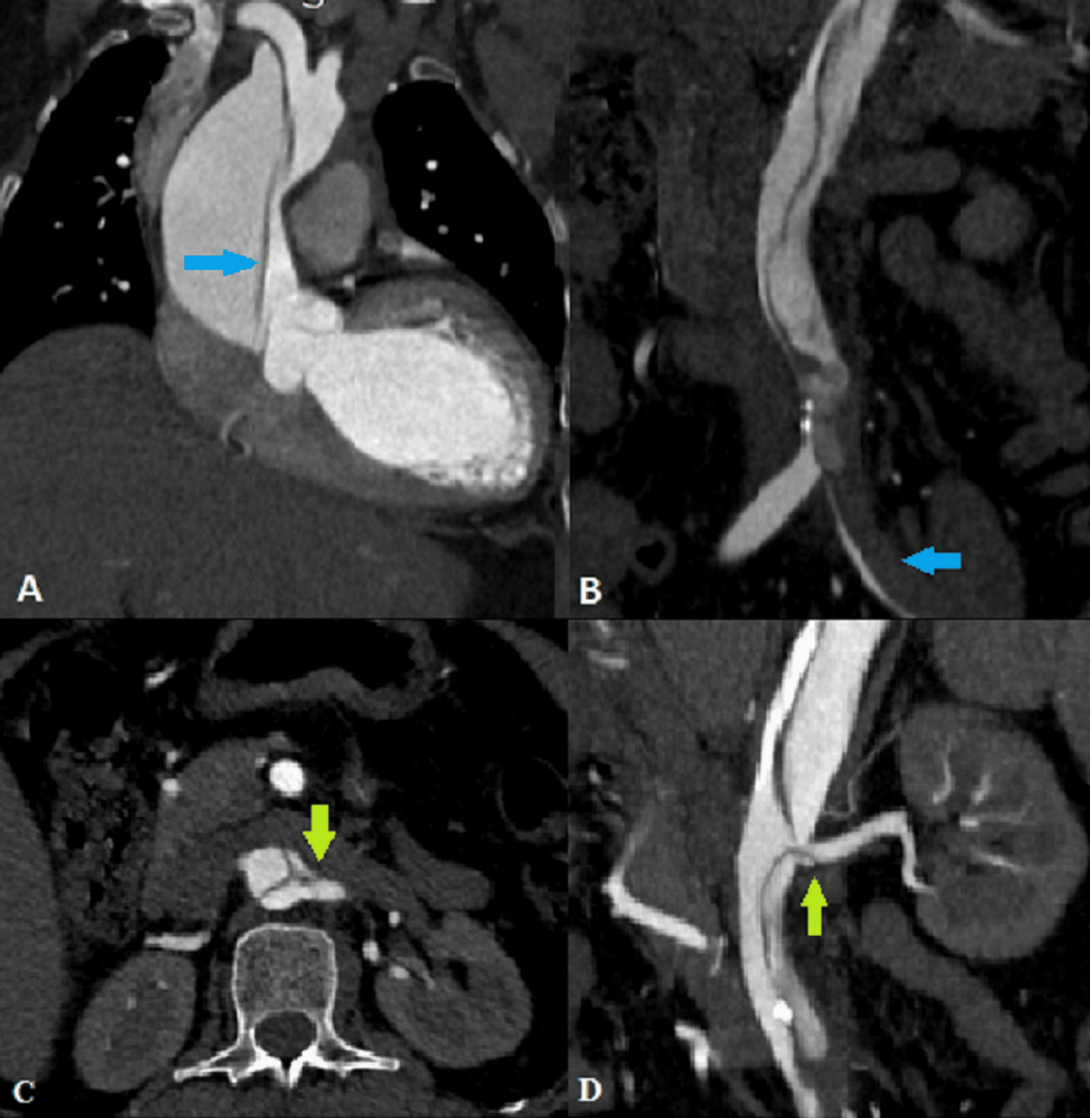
Coronal CT angiography images demonstrating (A) dissecting intimal flap in the ascending aorta (blue arrow) with differential enhancement of the true (smaller) and false (larger) lumens; (B) dissecting intimal flap extending into the abdominal aorta and left common iliac artery with thrombus in false lumen (blue arrow); (C) axial and (D) coronal CT angiography images demonstrating extension of intimal flap into the proximal left renal artery (green arrows).
